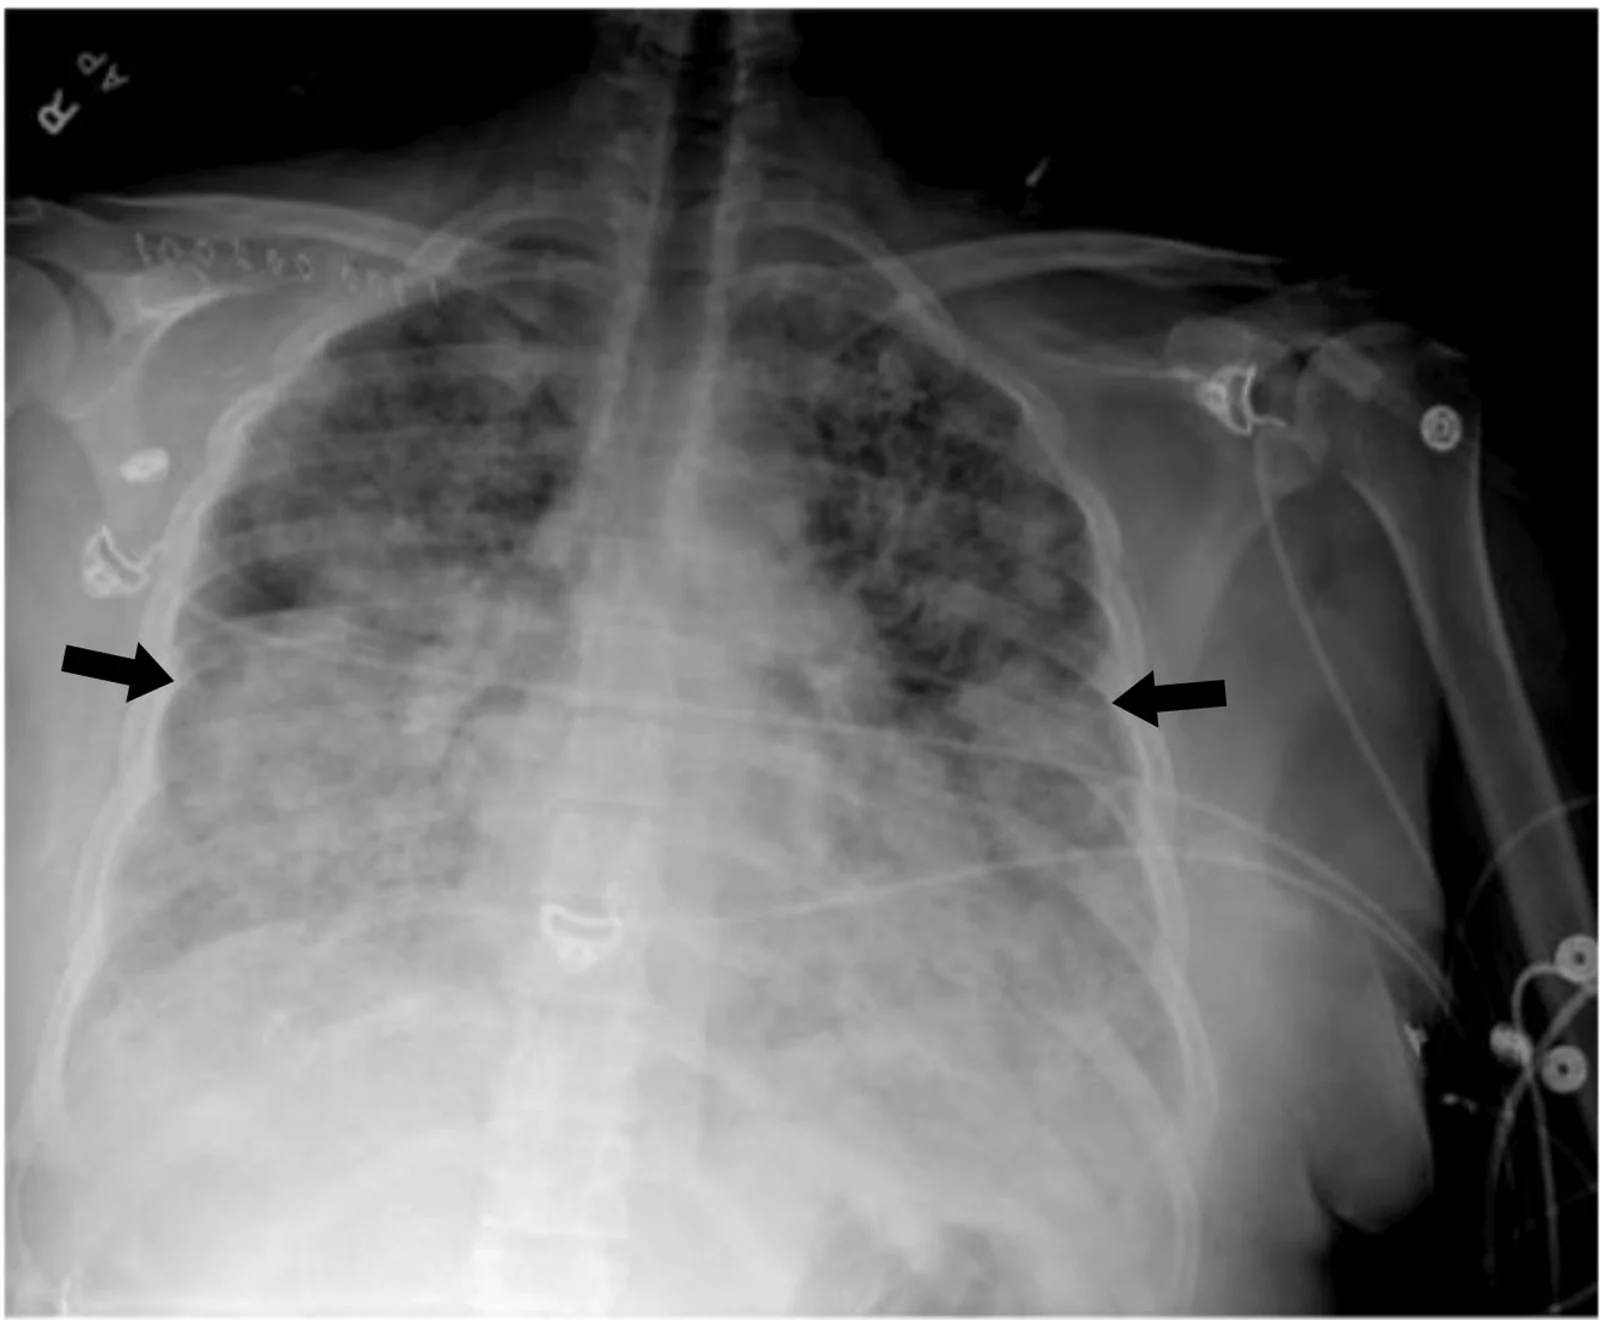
Chest X-ray demonstrating bilateral pulmonary opacities (black arrows) 14 days after patient's graft thrombectomy, status post eight units of packed red blood cells.
